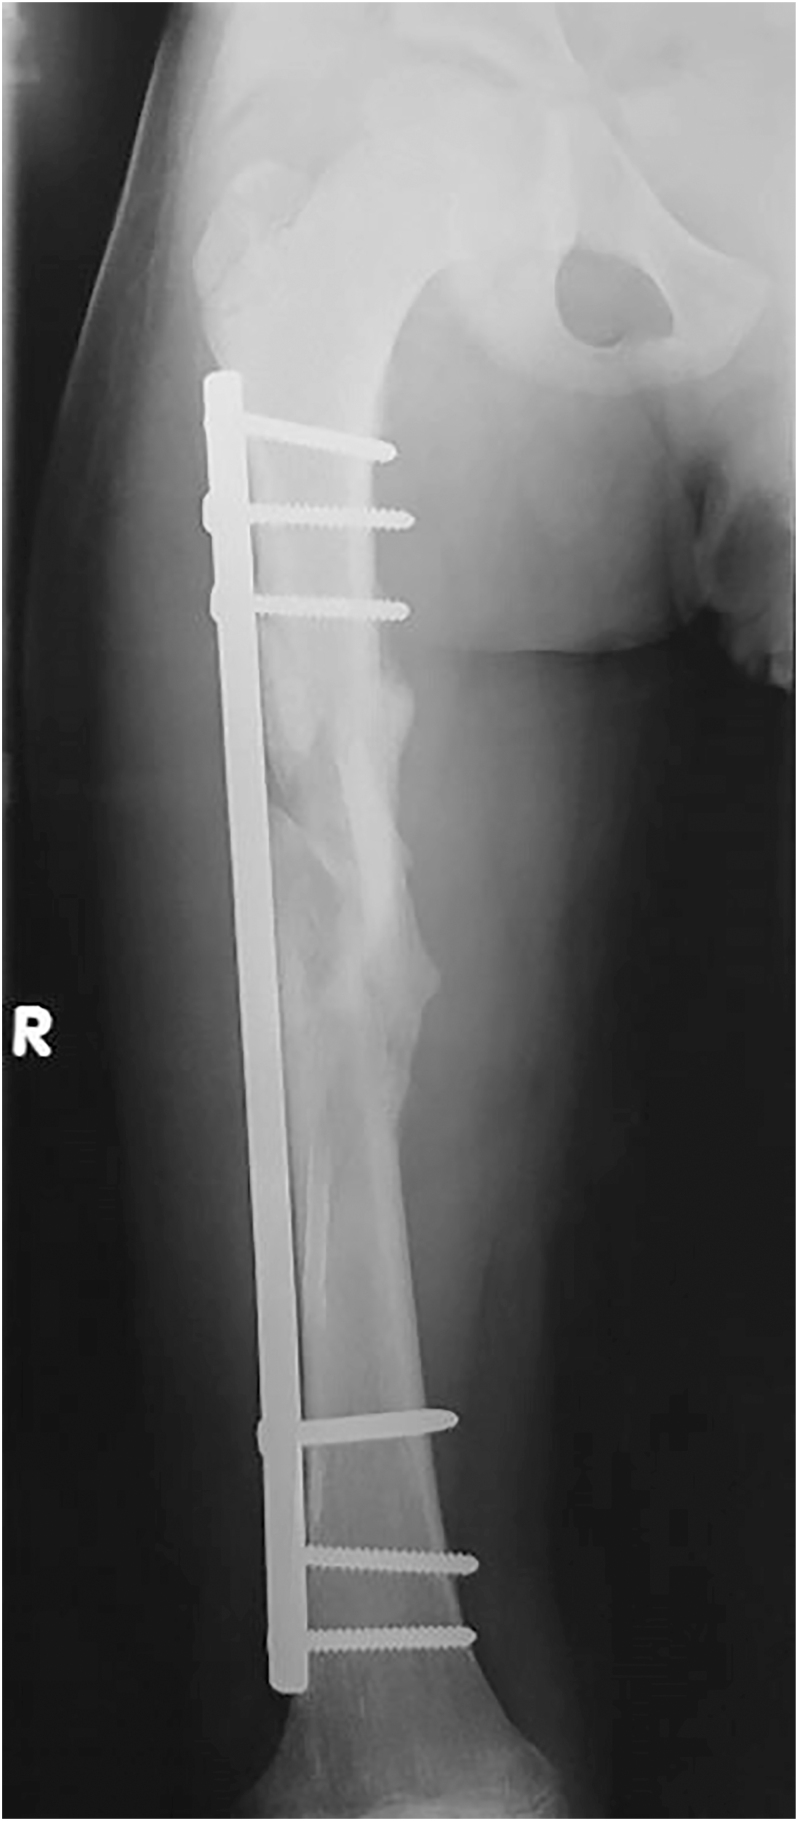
Radiograph showing a clustered screw pattern, with screws placed at both ends of the plate.
